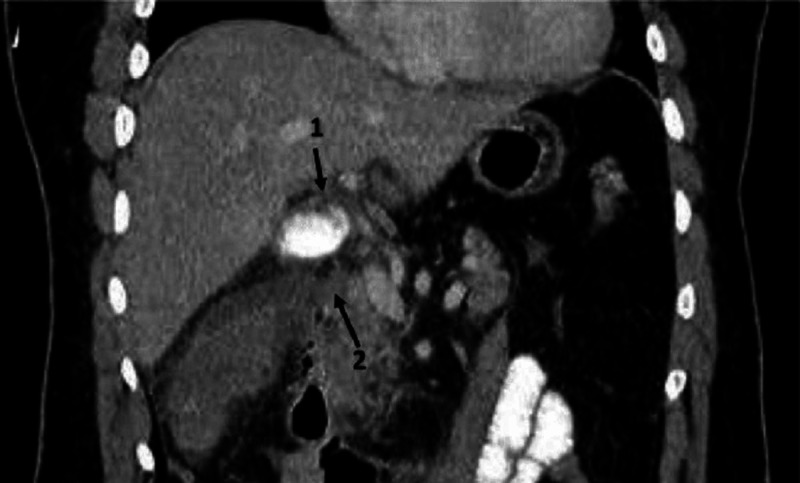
Coronal CT plane, showing extensive inflammatory changes and the thickening of gall bladder wall (1) with pericholecystic fat stranding (2)
